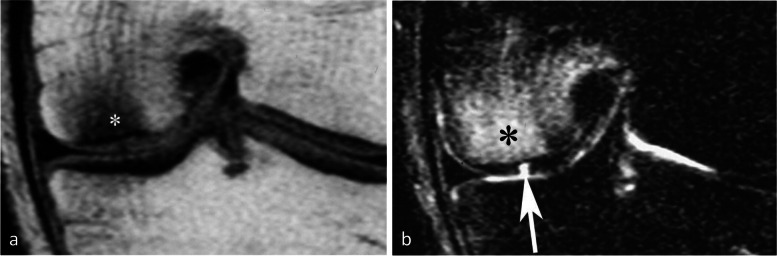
Bone marrow edema (BME)-like signal associated with focal chondral lesion. a, b T1-weighted (a) and fat-suppressed T2-weighted MR images (b) show BME-like signal intensities similar to those of subchondral insufficiency fractures (SIFs) (asterisks). Here, the BME-like signal intensity is related to a deep focal cartilage defect (arrow in b), evident in the present case
